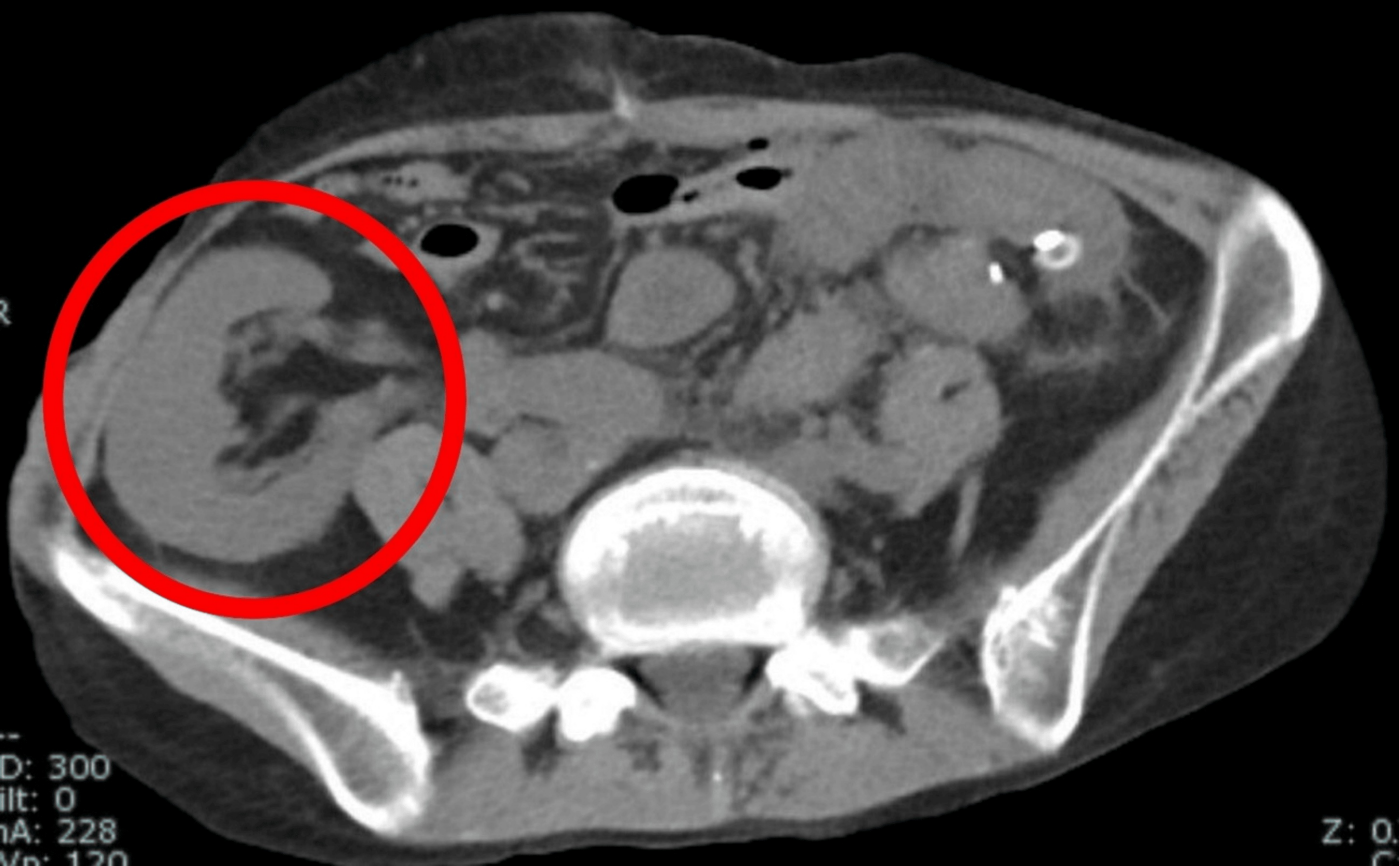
Preoperative abdominal CT (axial) showing transplanted kidney. Abdominal CT showing a transplanted kidney in the right iliac crest (red circle). CT, Computed Tomography
